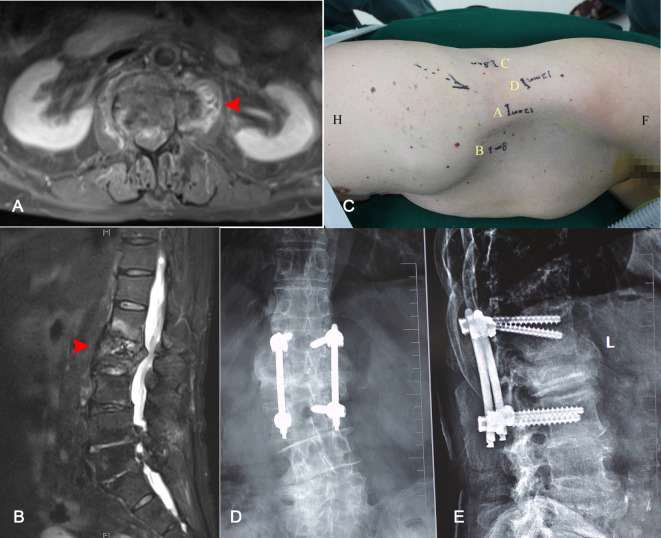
Cross section (A) and sagittal (B) T2-weighted MRI demonstrated destruction at the L1/2 intervertebral space and a partial L2 vertebral body (red arrow). The location of the working channel was planned before operation (C). Thirty months after the operation, the x-ray of the lumbar spine performed in the positive position (D) and the lateral position (E) showed the formation and fusion of the vertebrae.
